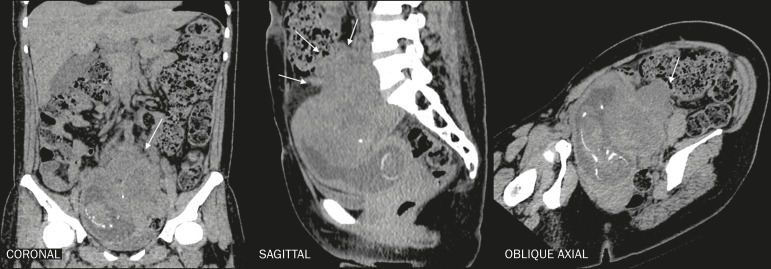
CT scan of a patient with a tumor in the organ of Zuckerkandl (arrows).
